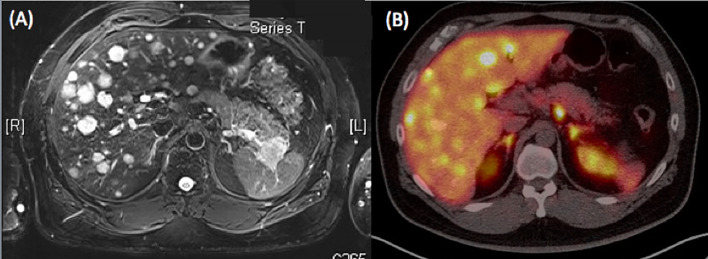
(A) MRI of the abdomen revealed a solid mass at the pancreatic tail with numerous liver tumors favoring metastases. The pancreatic body tumor was a cyst. (B) PET with MRI also showed peripancreatic lymph node metastases.
